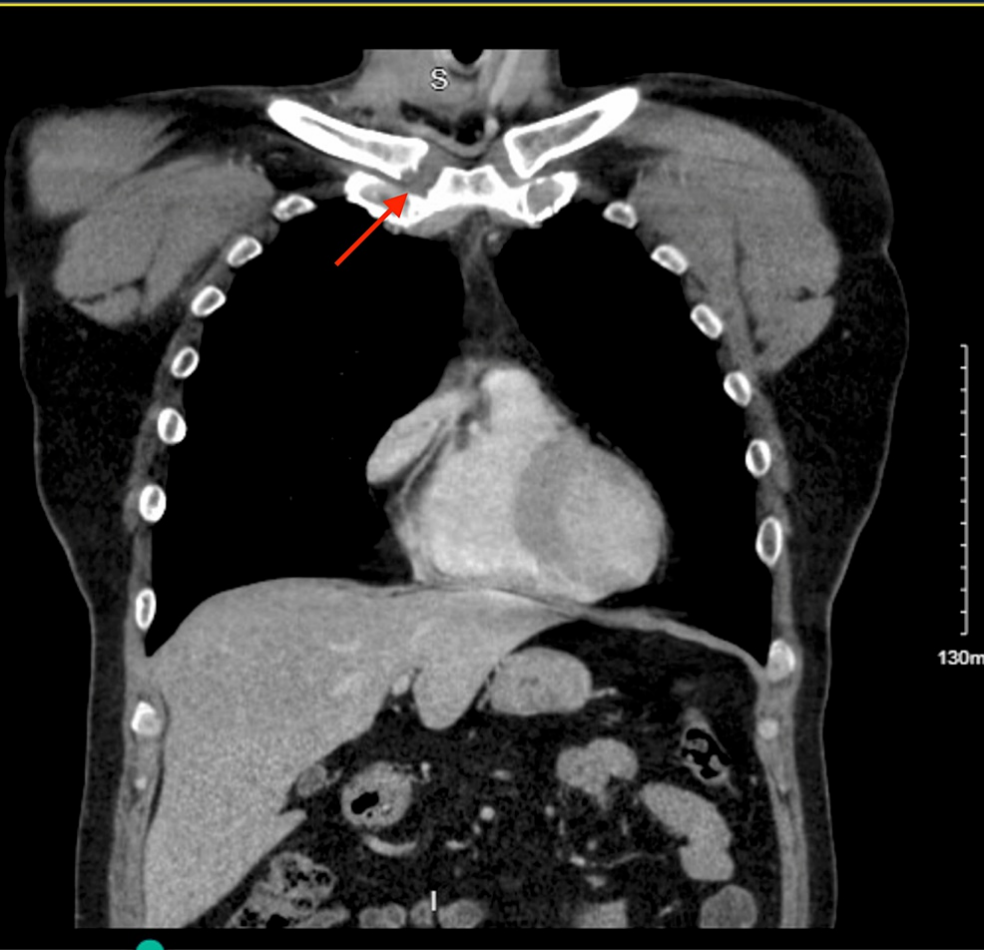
CT chest with IV contrast, coronal view: demonstrating induration and subcutaneous fat stranding at the right sternoclavicular joint with evidence of destruction of the cortical portion of the clavicle. There also appears to be increased soft tissue density within the joint.
